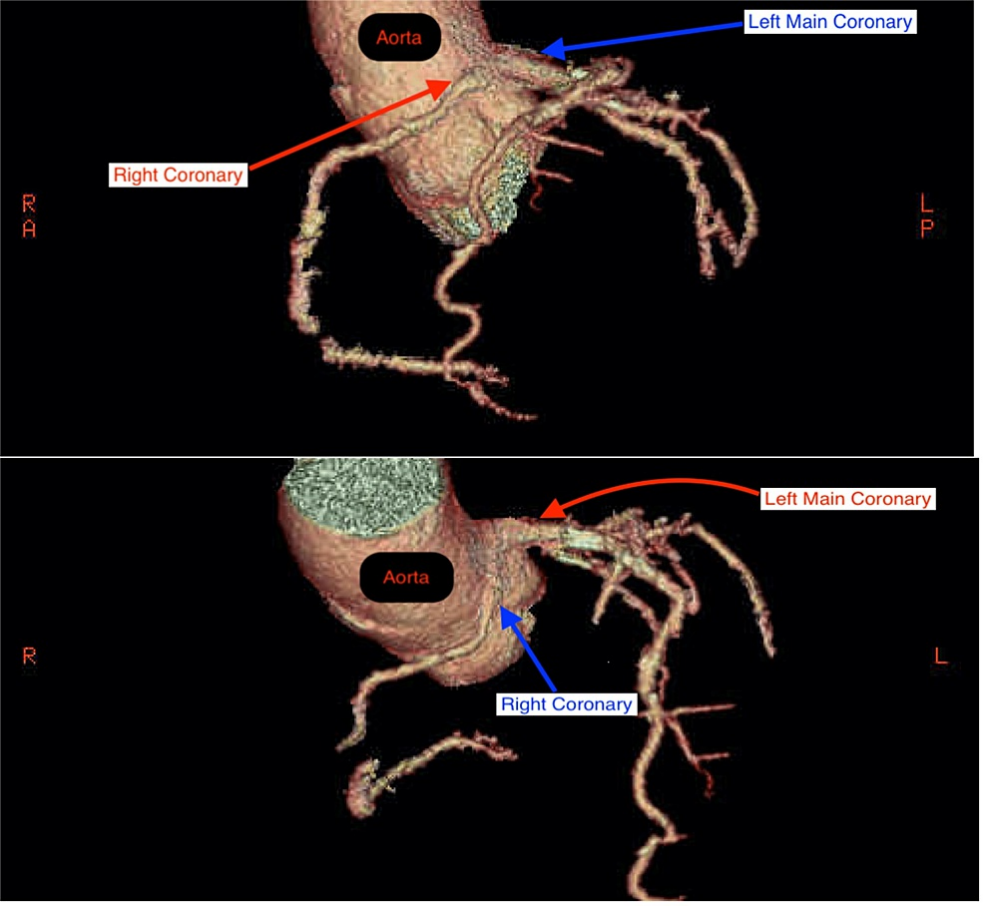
CCTA 3D reconstructed images identifying the anomalous RCA origin from the left aortic cusp and its surrounding structures. 3D: three-dimensional; CCTA: coronary computed tomography angiogram; RCA: right coronary artery
